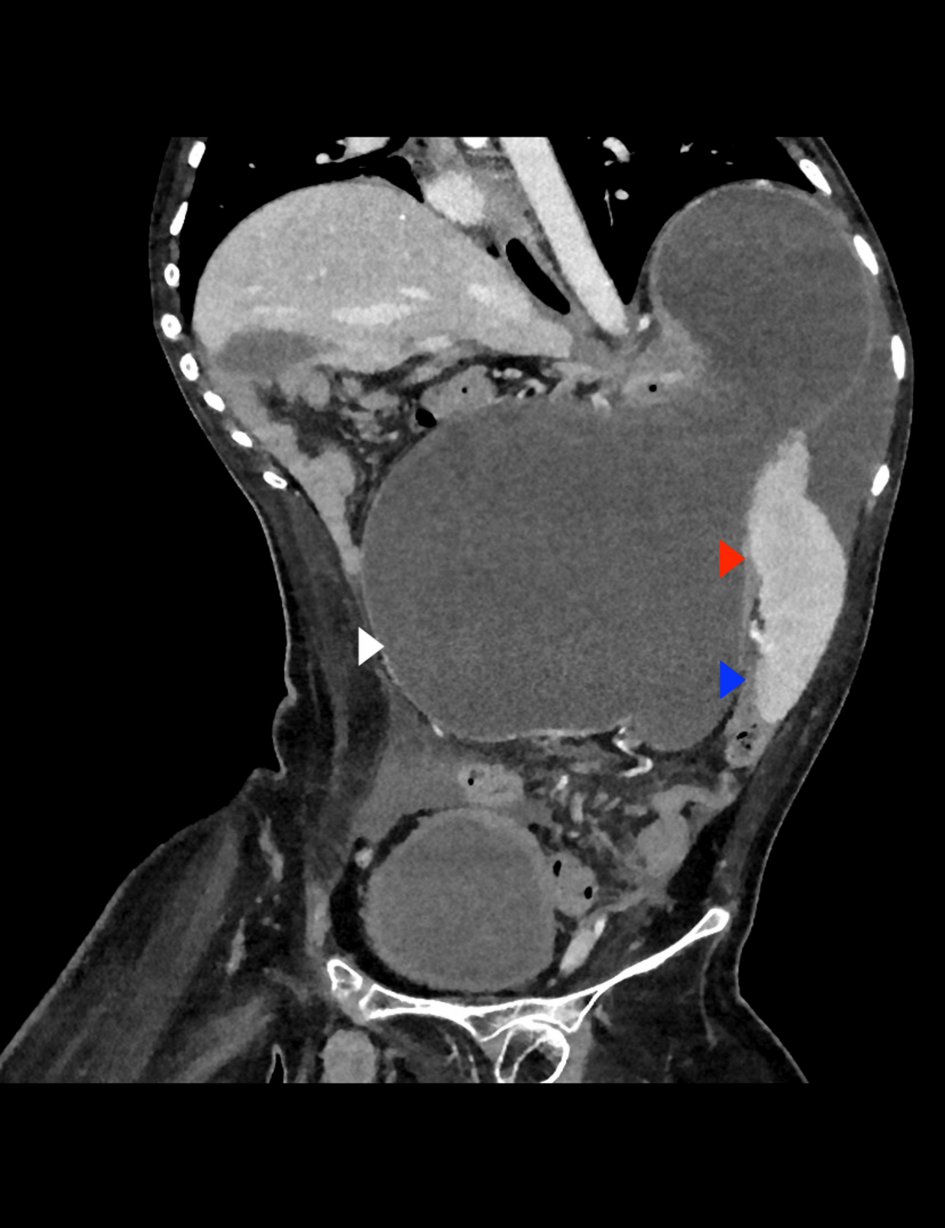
Computed tomography (CT) of the abdomen (coronal view) Severe gastric distention (white arrow) with a large fluid-air level (red arrow), a finding consistent with gastric outlet obstruction, is noted. A small amount of free fluid (blue arrow) is noted in the left peritoneal cavity. This finding is highly suggestive of gastric volvulus.
